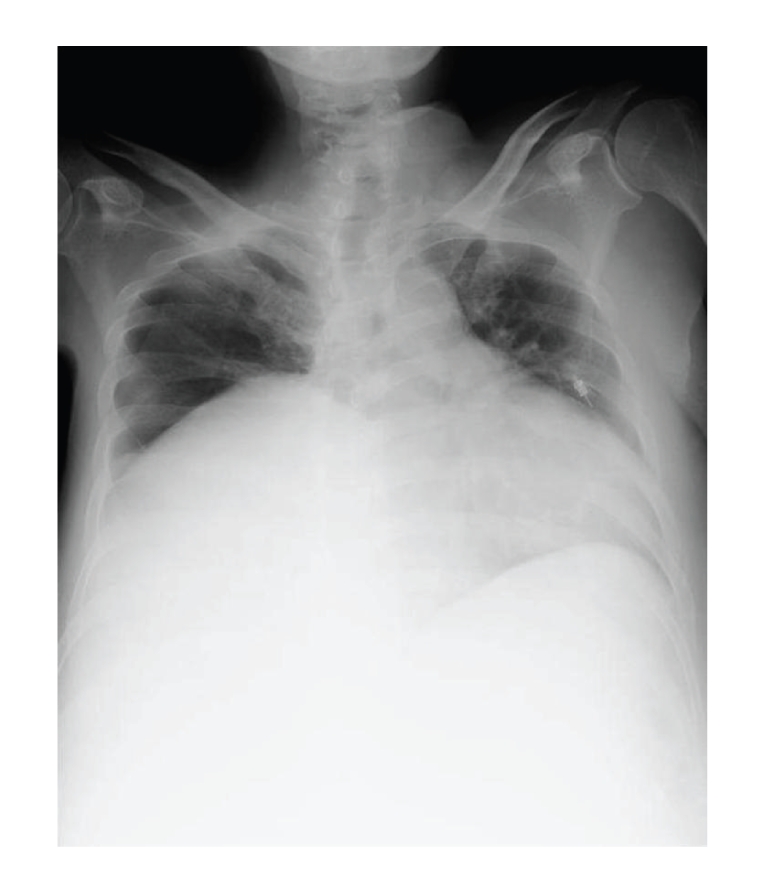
Chest X-ray showed that elevation of the diaphragm due to rapid growth of retroperitoneal tumor and cardiac enlargement.
